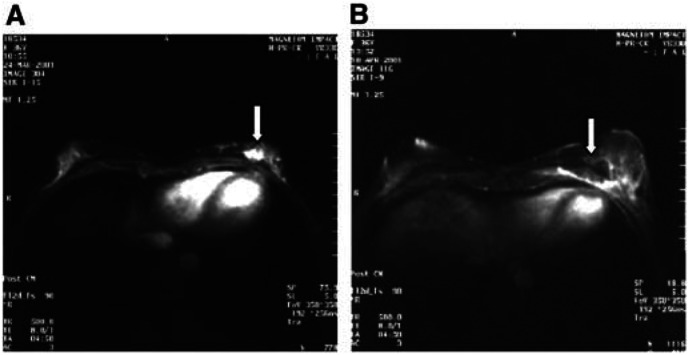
Contrast-enhanced MRI of a left breast cancer (arrowed) in a 36-year-old woman before (A) and 1 week after (B) HIFU ablation. Note the absence of contrast uptake in ablated volume, including tumour and a margin of treated normal breast tissue about 1.5–2.0 cm around the cancer. Oedema is observed in the mammary tissue surrounding the treated region.
